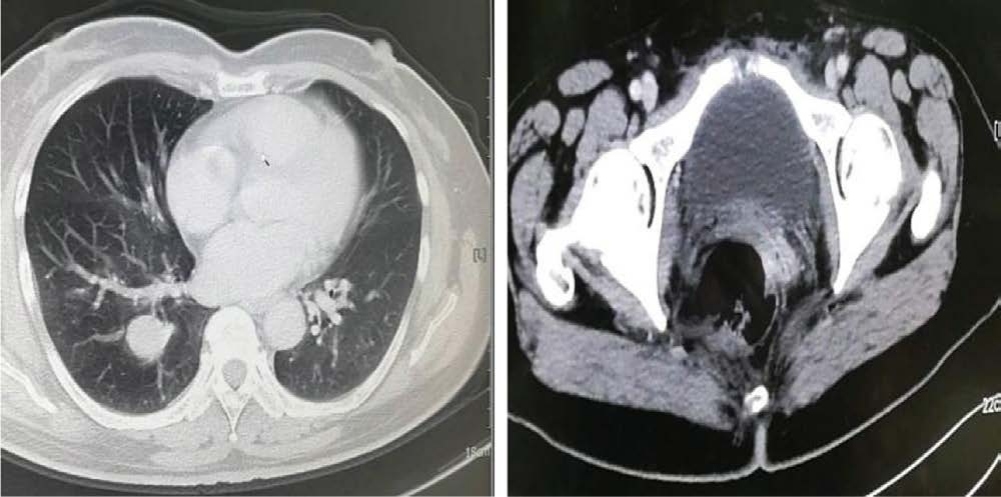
Computed tomographic scan examination revealed relapse in lung and pelvis.
